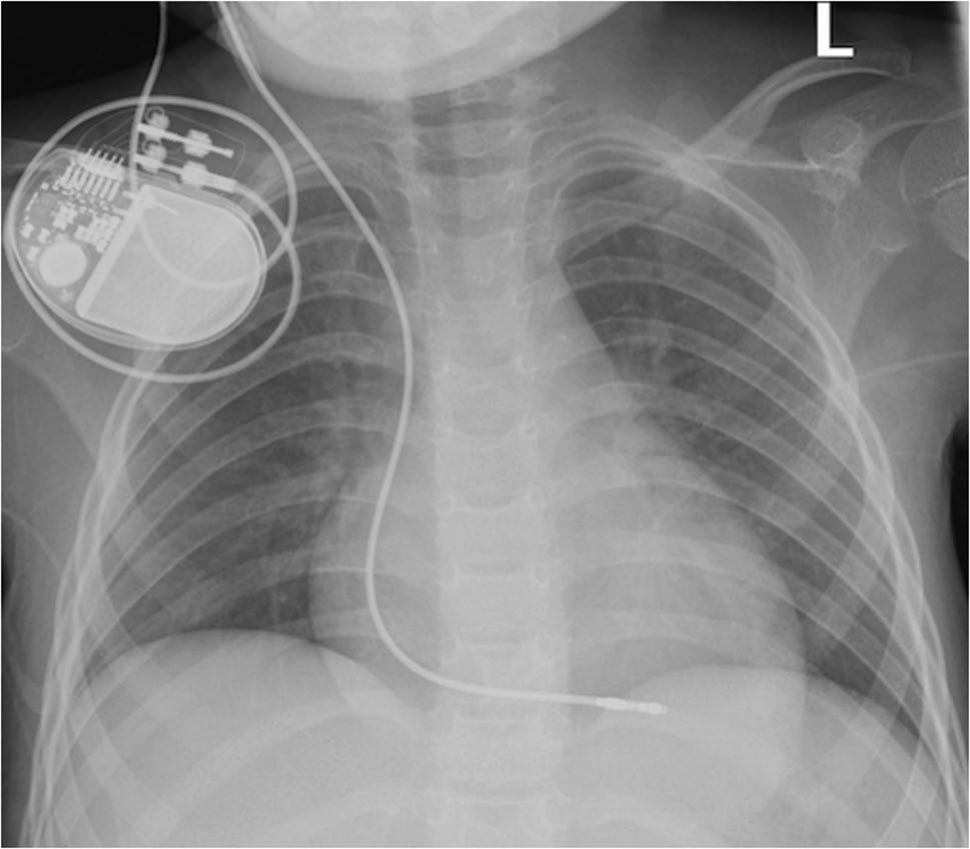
A 6-year-old girl presented with bradycardia secondary to type II Mobitz and intermittent complete heart block. Anteroposterior chest radiograph shows the appropriate placement of the transvenous pacing lead, with its tip in the right ventricle and the externalized pacemaker generator taped to the skin
